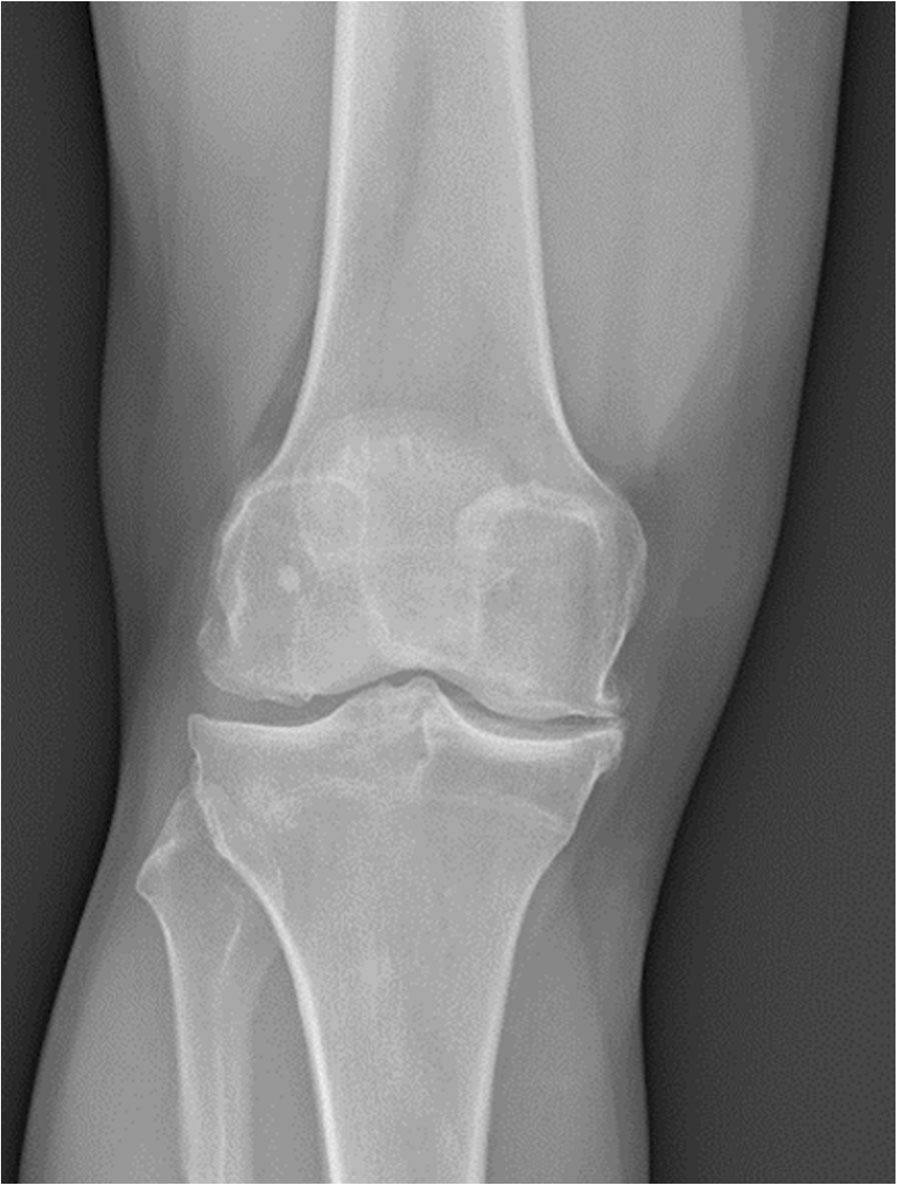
Pre-operative AP knee radiography Patient 1
